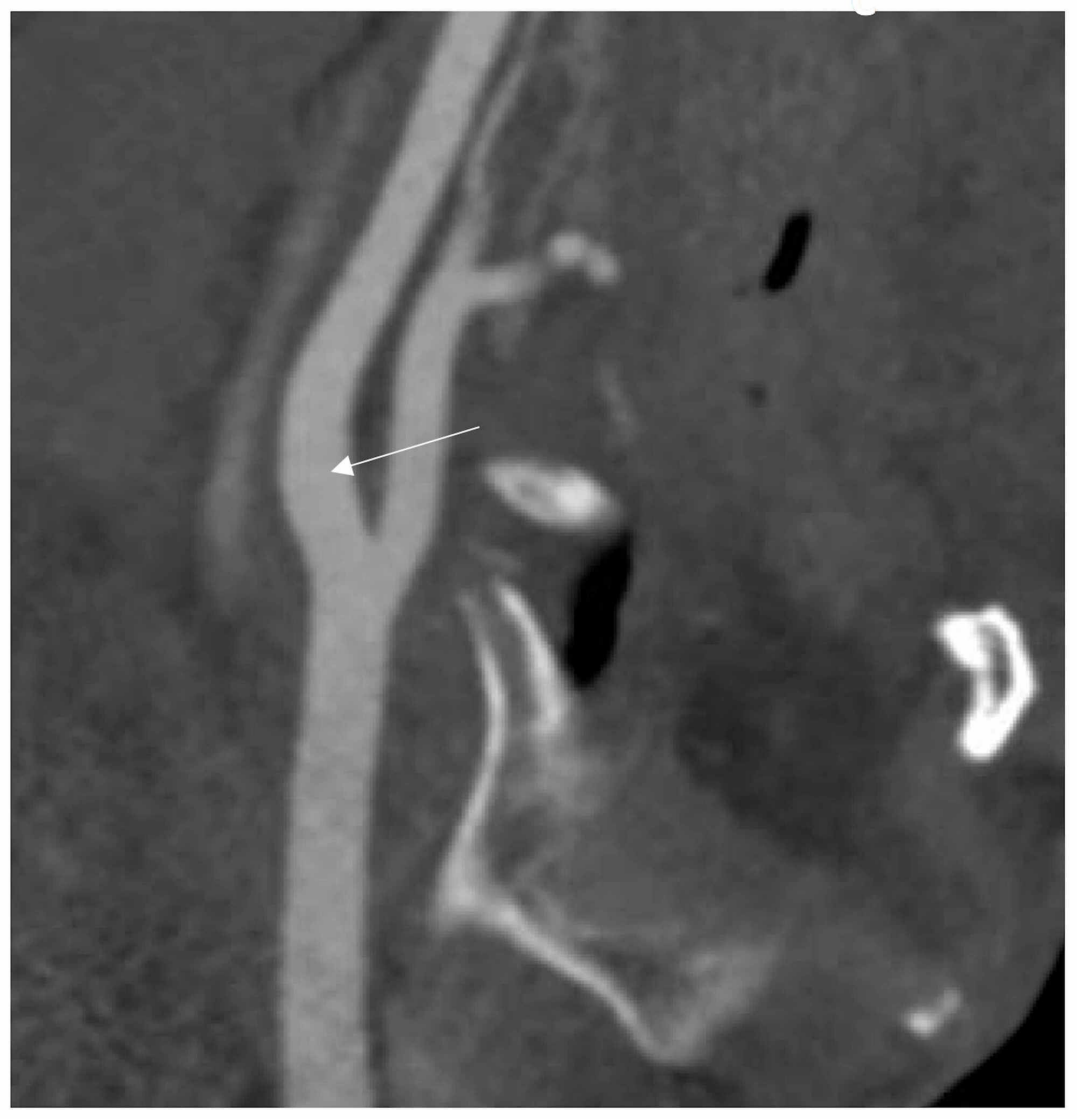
Computed tomography angiography follow-up of case 2 Computed tomography angiography sagittal view on 14-day follow-up showing complete resolution of the carotid plaque
